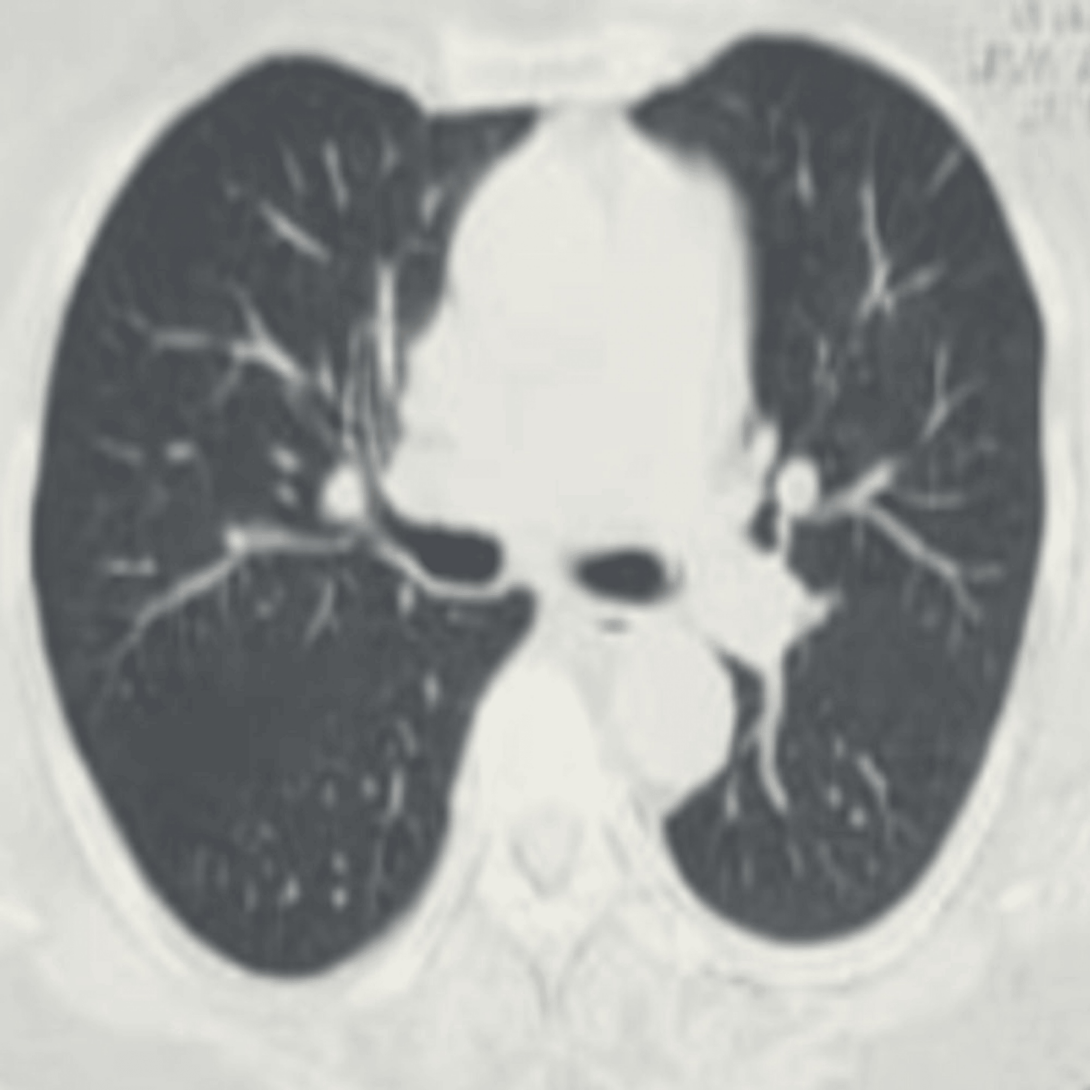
Transverse computed tomography of the lung window reveals at the end of course of steroid showing complete resolution of miliary opacity
